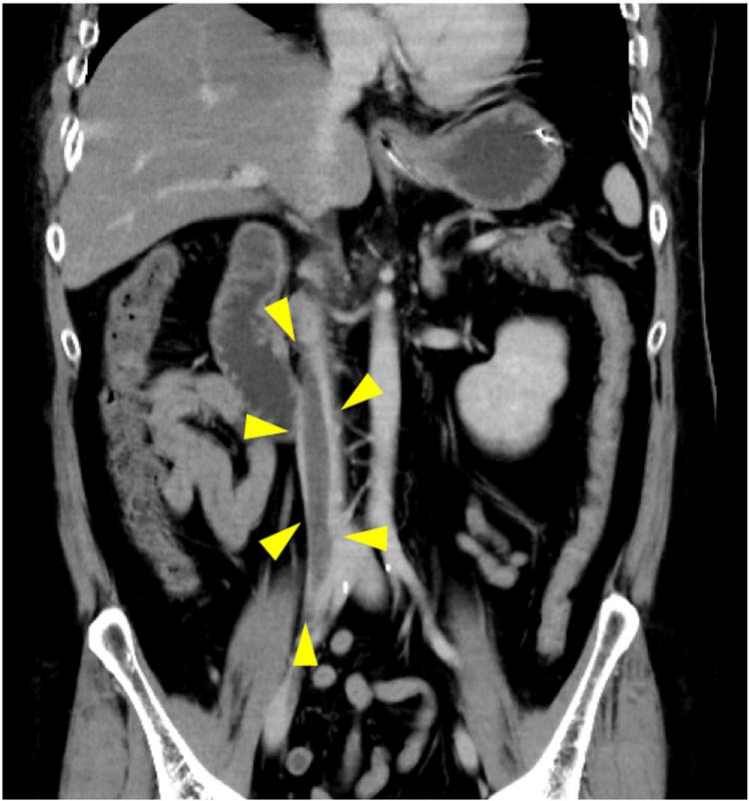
Contrast-enhanced computed tomography (coronal view) on admission. A thrombus (yellow arrowheads) is located in the inferior vena cava from the right iliac vein.
